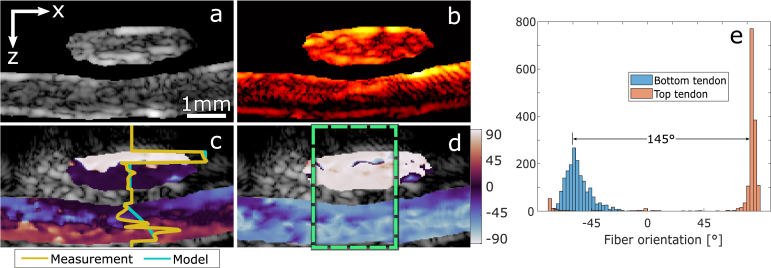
Analysis of tendons with a relative orientation of 30° between them. (a) is the ultrasound image, and (b) is the mean PA image. (c) is the phase detection for the two layers. The profile plot of the phase shows a progressive variation caused by the phase difference between the built-up fluence from the first layer and the absorption being much smaller than 90°. (d) is the corrected detection of fibers’ orientation. (e) shows the histograms for the two tendons within the region indicated by the dashed square.
